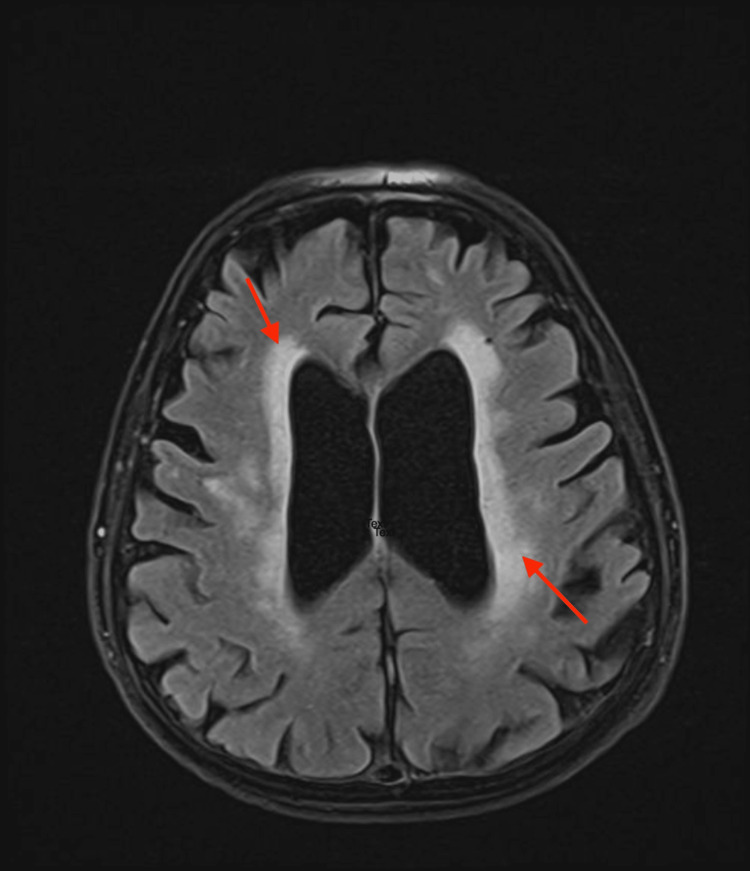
Axial FLAIR MRI of the brain showing periventricular white matter changes Axial FLAIR MRI demonstrates bilateral periventricular hyperintense lesions surrounding the lateral ventricles (arrows), consistent with chronic small vessel ischemic changes. The ventricles appear mildly enlarged with prominence of cortical sulci, suggestive of cerebral atrophy. No evidence of acute ischemia. FLAIR, fluid-attenuated inversion recovery
